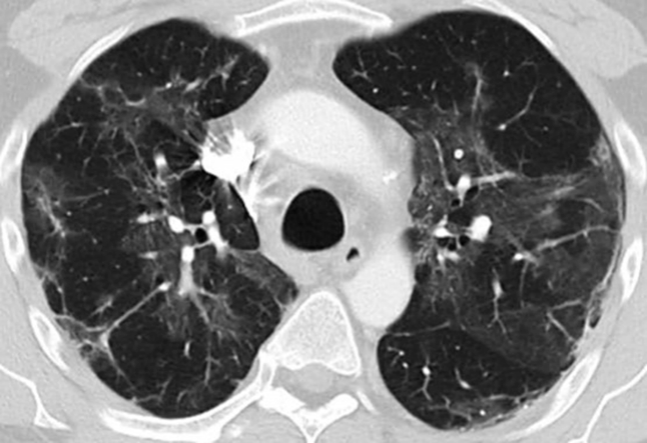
Lung CT scan in the upper lobe 4 weeks after symptom onset showing patchy subpleural ground glass opacities and linear consolidation
